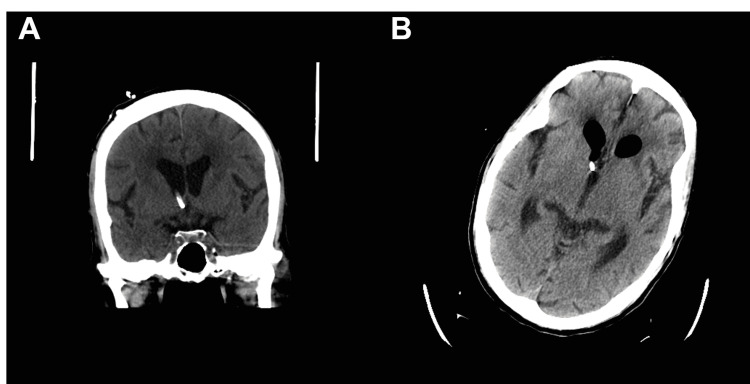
Coronal (A) and axial (B) CT of the brain without contrast showing proper placement of an external ventricular drain.
